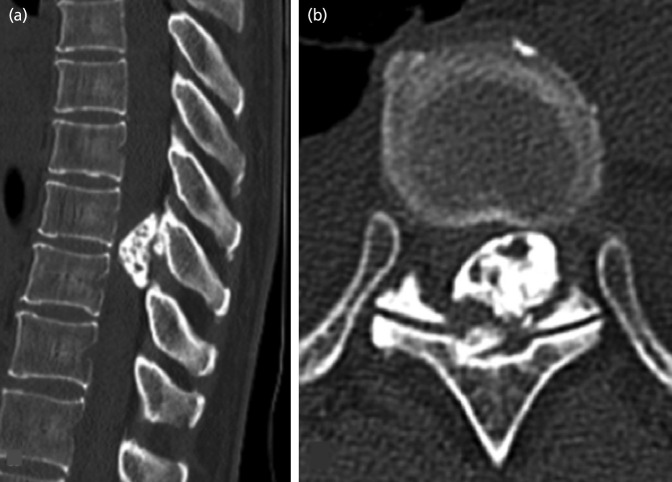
Case 9. Plain CT showing osseous lesion in continuity with the lamina and protrusion into the spinal canal. (a) sagittal image, (b) axial image.
